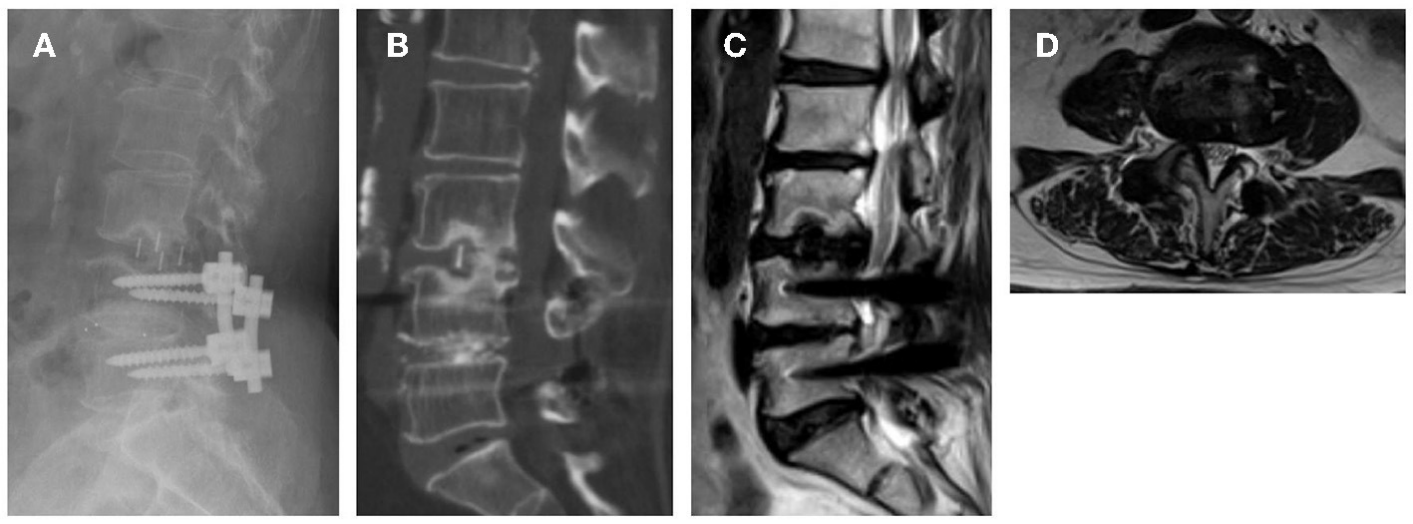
Images obtained in a 66-year-old woman who had an L4–L5 posterior instrumented fusion 26 month ago. She experienced new leg pain and intermittent claudication due to adjacent-segment degeneration and stenosis for 2 months. (A) 18 months follow-up reviewed Grade I subsidence; (B) CT confirmed bony fusion was achieved; (C,D) Indirect decompression of spinal canal was maintained.
